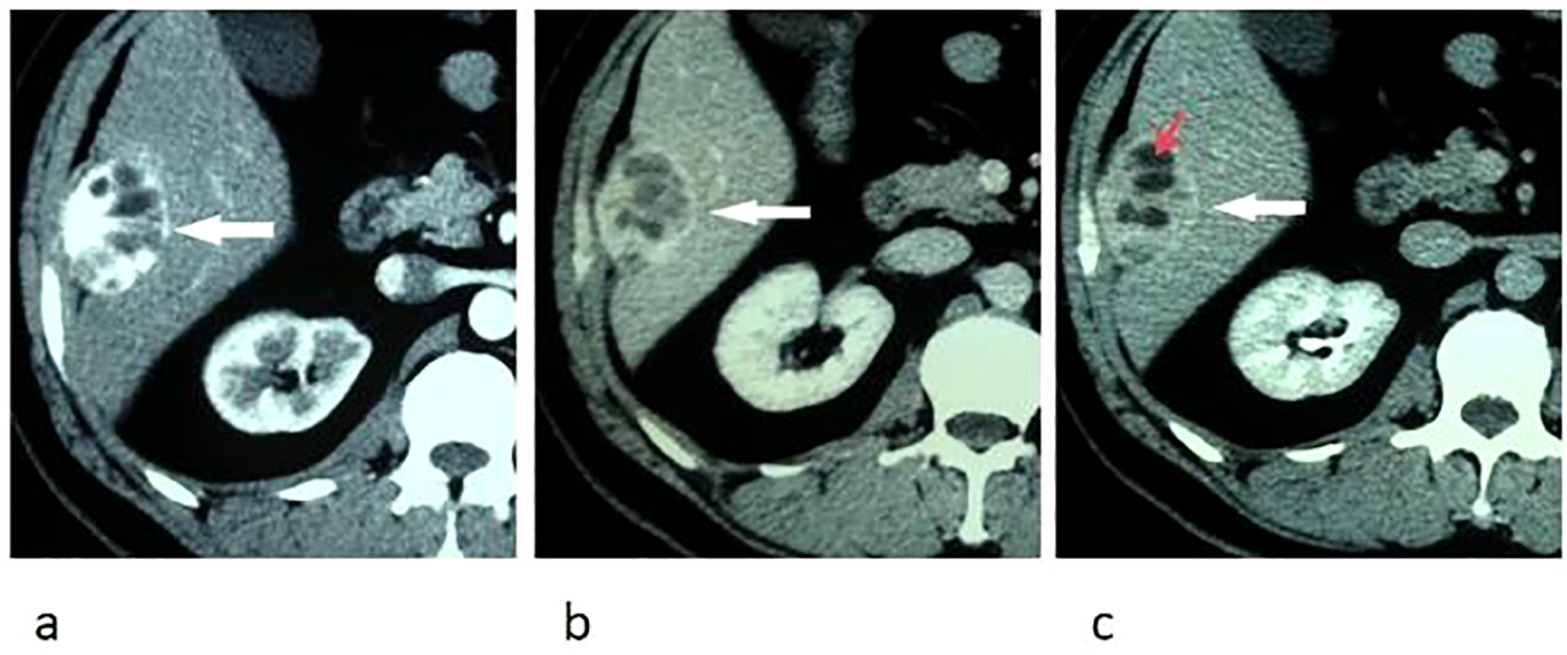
Transverse CT images of arterial phase (a), portal venous phase (b), and delay phase (c) show marked and prolonged enhancement of an oval liver mass, with unenhanced intratumoral cystic areas (red arrow) and enhanced capsule (white arrow).
